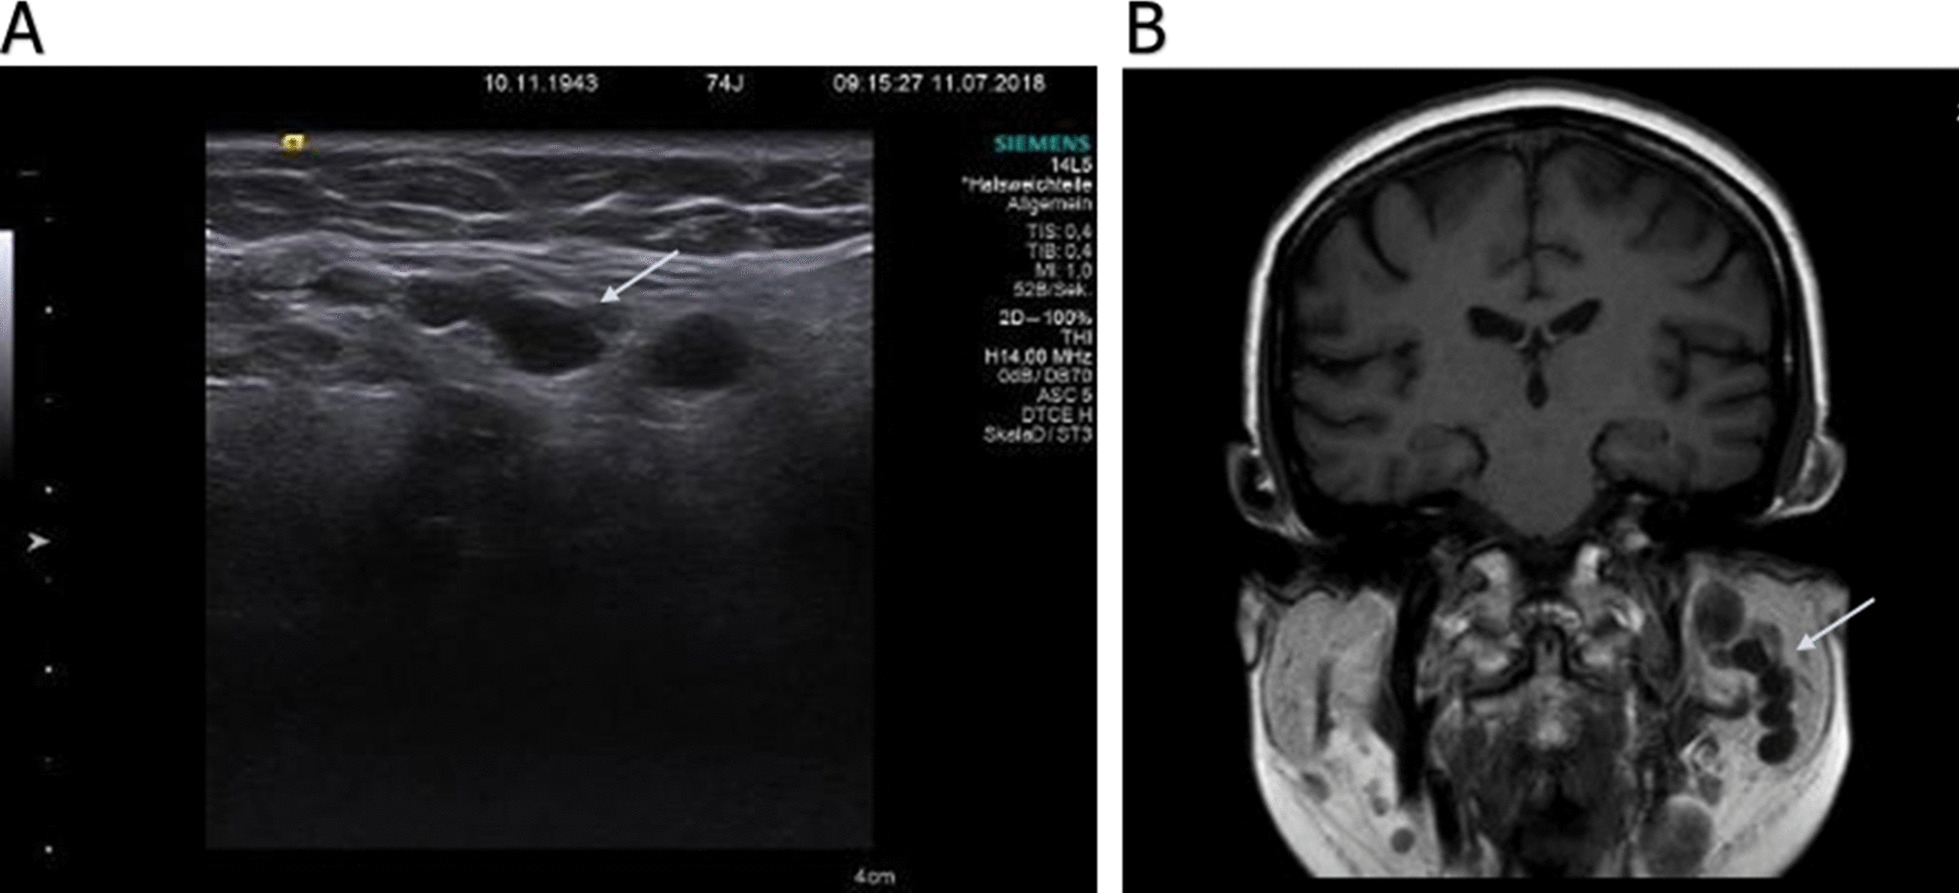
A Ultrasonography. Multiple round-shaped, homogeneous and smooth structures along the course of the left marginal mandibular branch of the facial nerve. B Cranial magnetic resonance imaging T1 coronal view. Multiple tumors in the left parotid gland along the facial nerve. A & B: Arrow is showing the tumors
